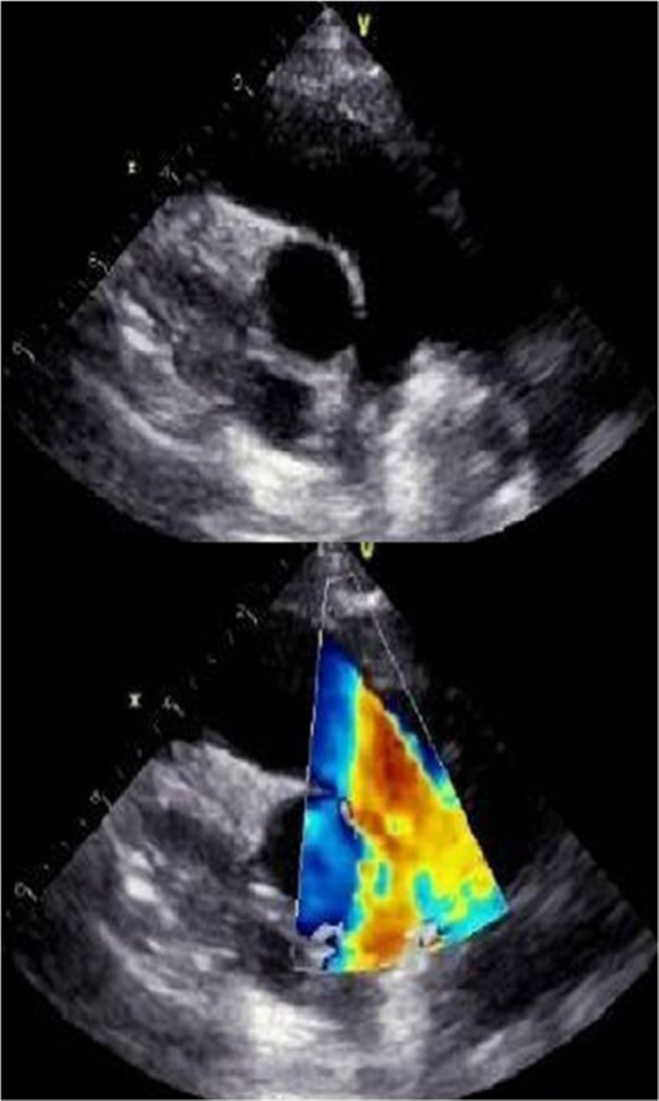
Postoperative echocardiography. Parasternal short-axis view at the level of the aortic valve showing an unobstructed right ventricular outflow tract.
